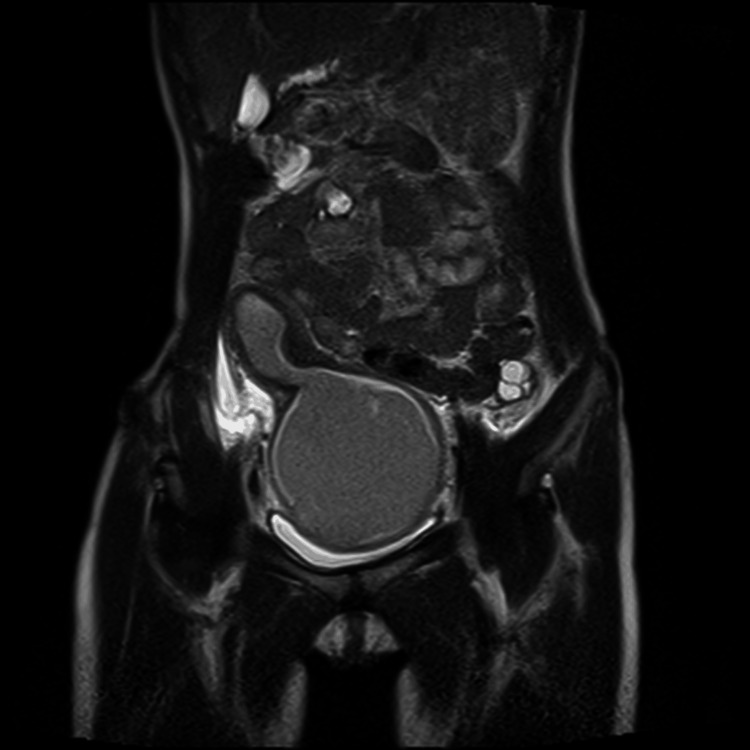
Predrainage MRI findings. On magnetic resonance imaging, a malformed uterus with intraluminal blood accumulation was observed. The enlarged uterus appears to be a contiguous adnexal horn originating from the main body of the uterus.
